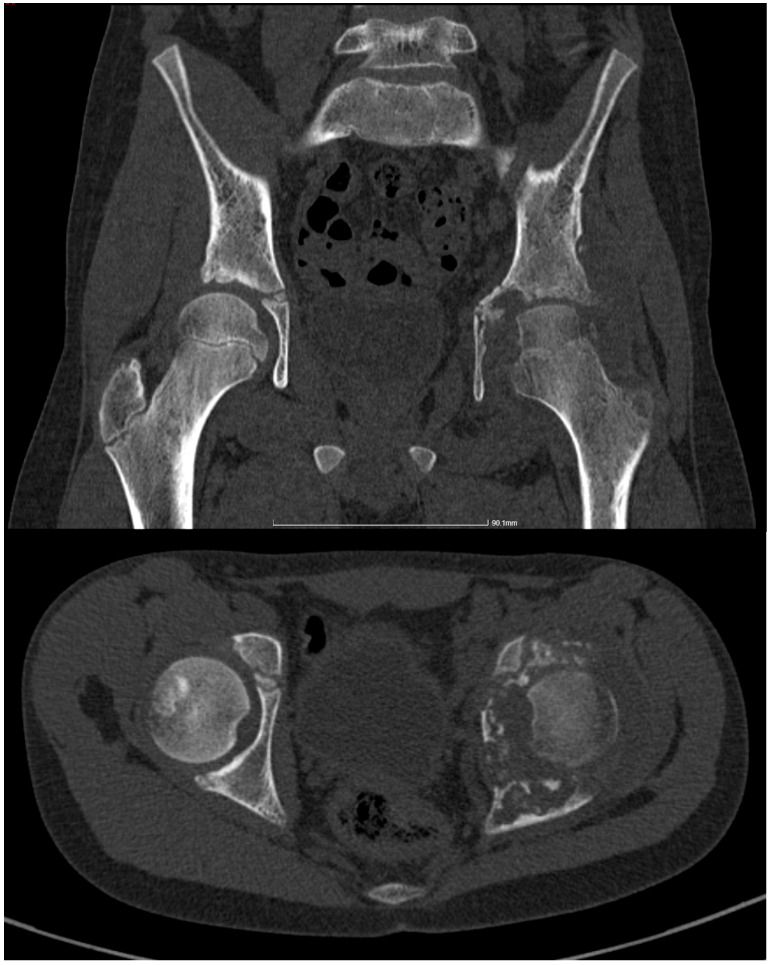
CT scan of the pelvis, cross-sectional and frontal views: extensive area of osteolysis and fragmentation, slightly expansive at the left acetabular and periacetabular level; multiple areas of osteolysis at the left femoral head with no periosteal reaction in the vicinity; intra-articular overflow in the left hip joint of approximately 15 mm, with parafluid densities and calcifications in the joint capsule, in the upper portion.
